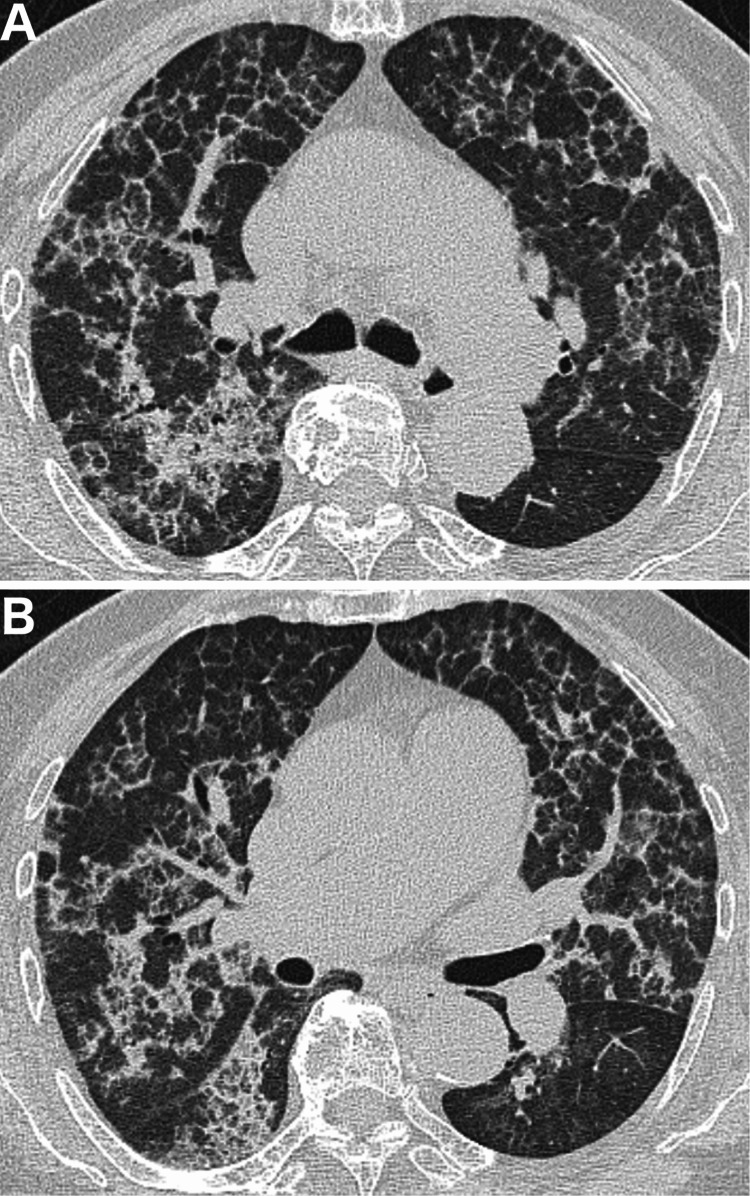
Chest CT scan at the time of diagnosis Chest computed tomography (CT) revealed interlobular septal thickening against a background of ground-glass opacities, presenting a crazy-paving pattern. A. CT slice at the carinal level. B. CT slice at the middle lobe and lingular level.
